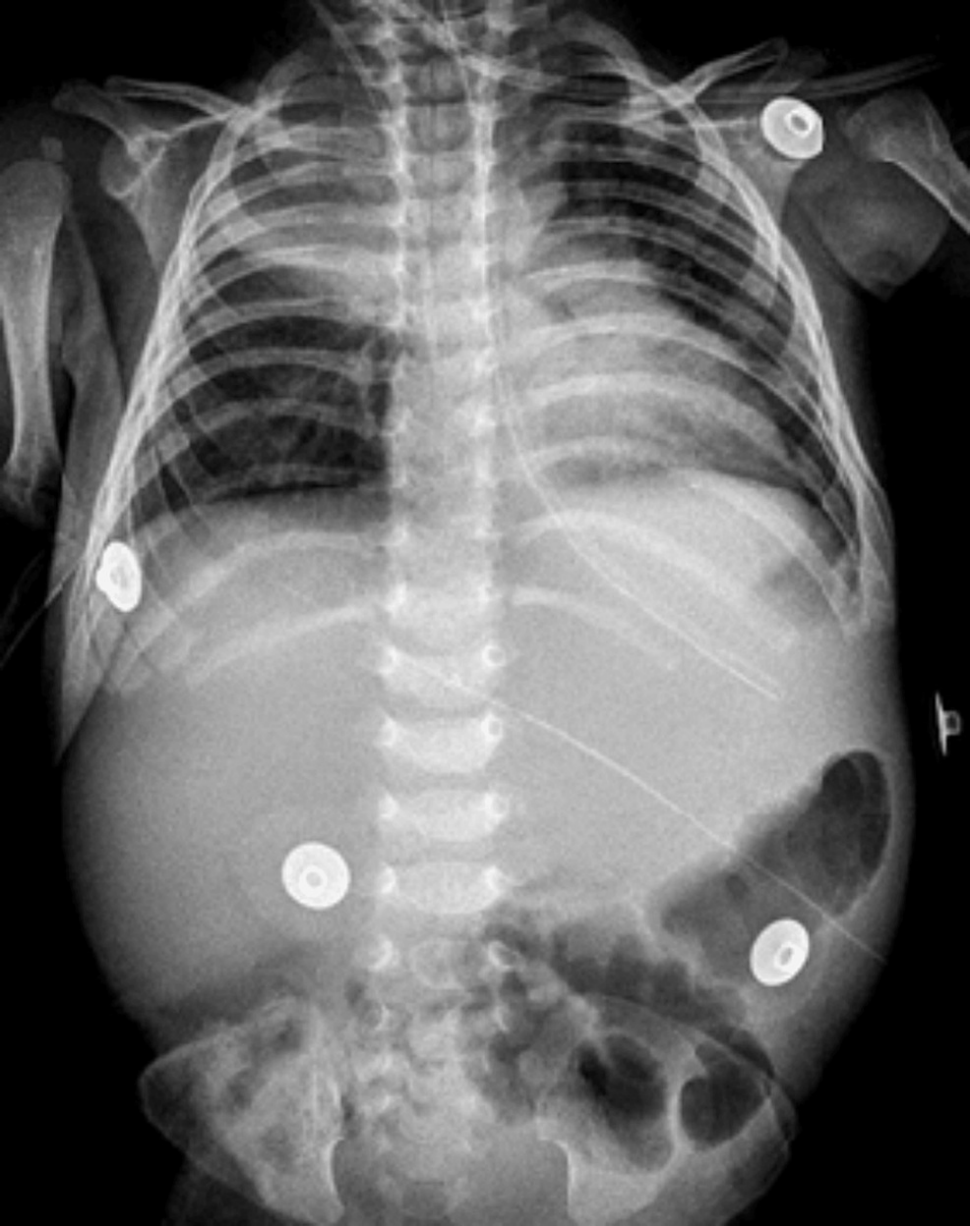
Plain chest and abdominal radiograph frontal view showing non-homogeneous opacities in the right upper lung zone with prominent pulmonary vasculature and enlarged hepatic silhouette with displacement of bowel loops
